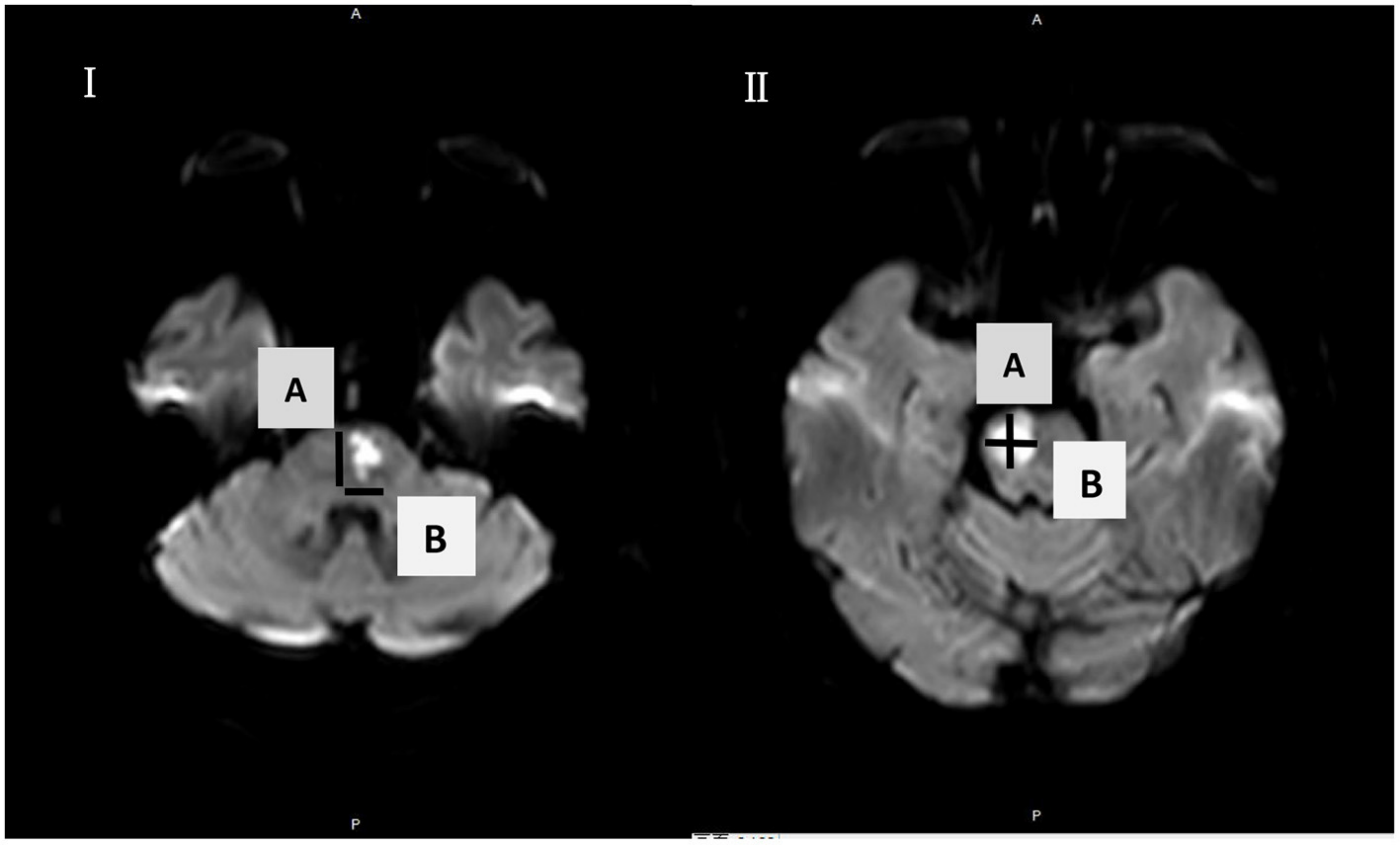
Acute pontine infarctions on axial diffusion-weighted imaging are shown in I and II. The maximal ventrodorsal (A) length and (B) width of each infarct were measured on axial MRI. A × B was used to define the infarction size (IS).
